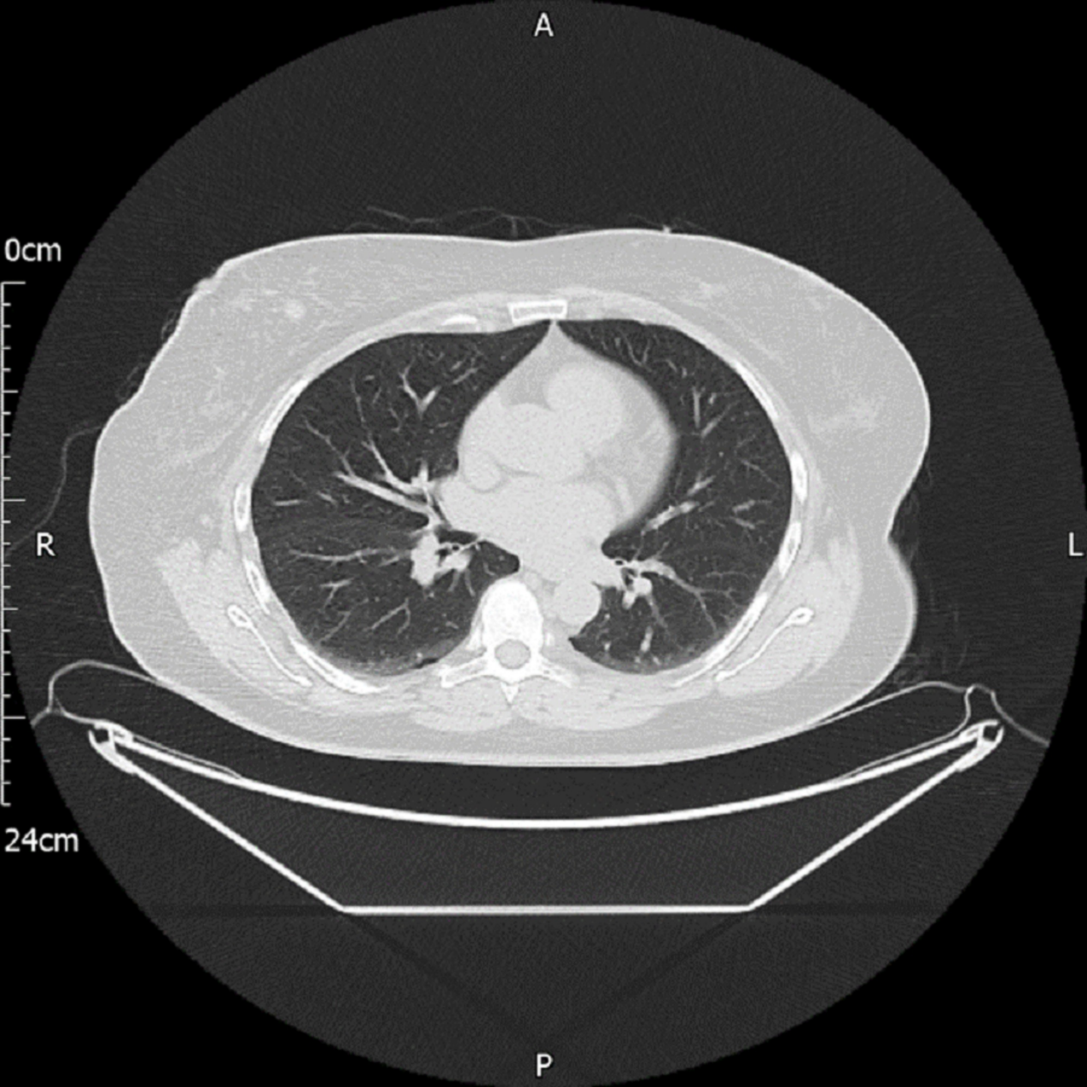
Chest computed tomography showing a small lesion in the right breast
